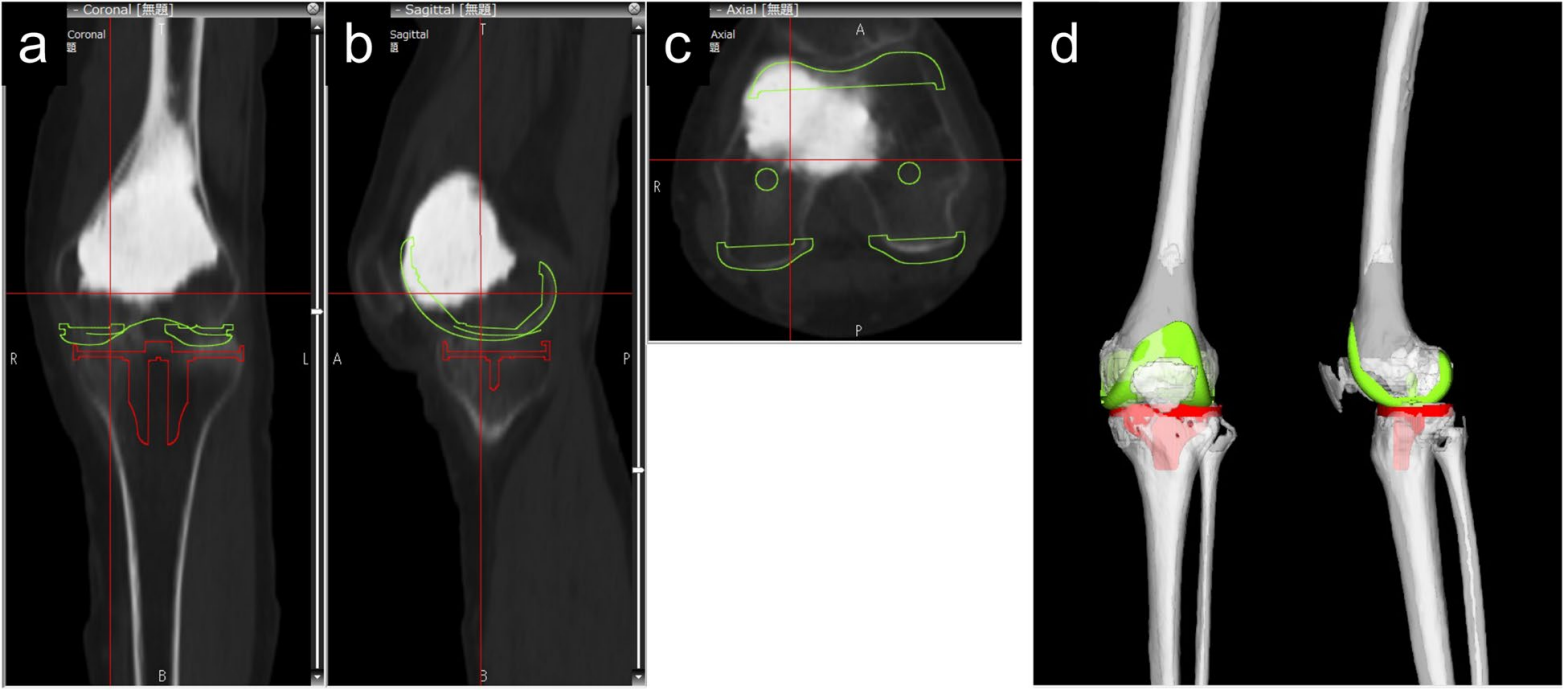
Three-dimensional templating imaging (a: coronal, b: sagittal, c: axial, d: 3D view) for computed tomography-free navigation assisted-surgery. The distal anterior part of calcium phosphate cement needed to be resected (b, c). The size and alignment of the component were three-dimensionally visualized (d)
